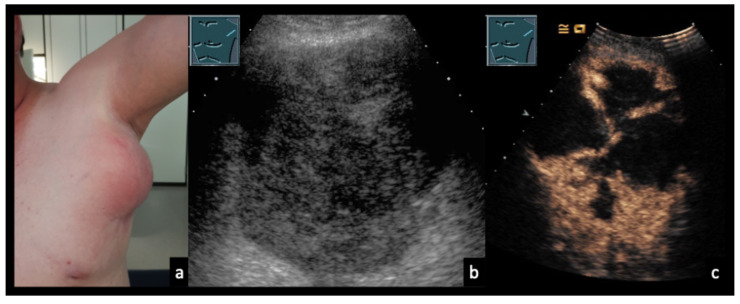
67-year-old male patient (a) with confirmed left axillary lymph node metastasis from malignant melanoma. (b) B-mode ultrasound shows an enlarged, heterogeneous lymph node. (c) On CEUS, there is partial absence of enhancement, consistent with necrotic transformation.
